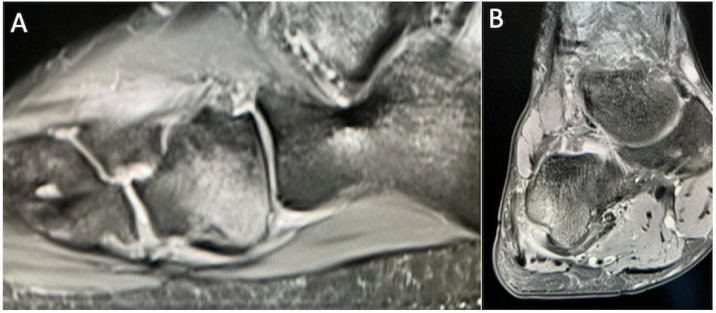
Sagittal (A) and coronal (B) STIR MRI demonstrating florid osseous oedema of the cuboid, secondary to peroneus longus tendinopathy.
